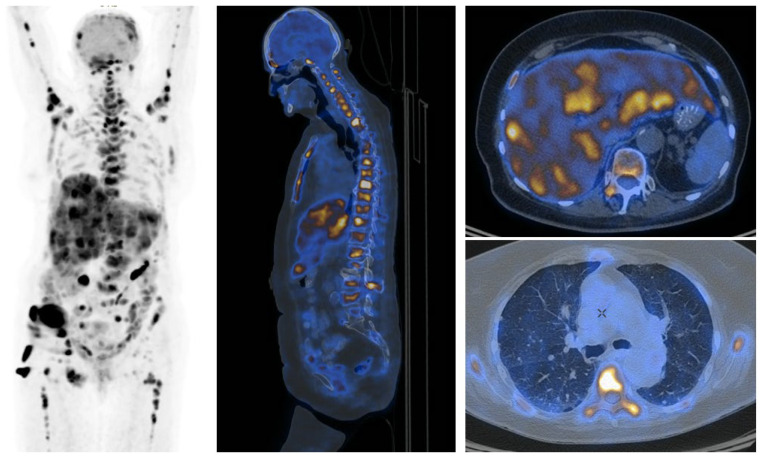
[^18^F] FDG PET/CT scan shows a 73-year-old woman with bladder cancer post-cystectomy. A dose of 4 MBq/kg was administered, and imaging was performed approximately 60 min after tracer injection. Multiple metastases are observed in the bones and liver with high [^18^F]FDG uptake. Additionally, multiple metastases, up to 8 mm in size, are detected in the lungs without tracer uptake.
